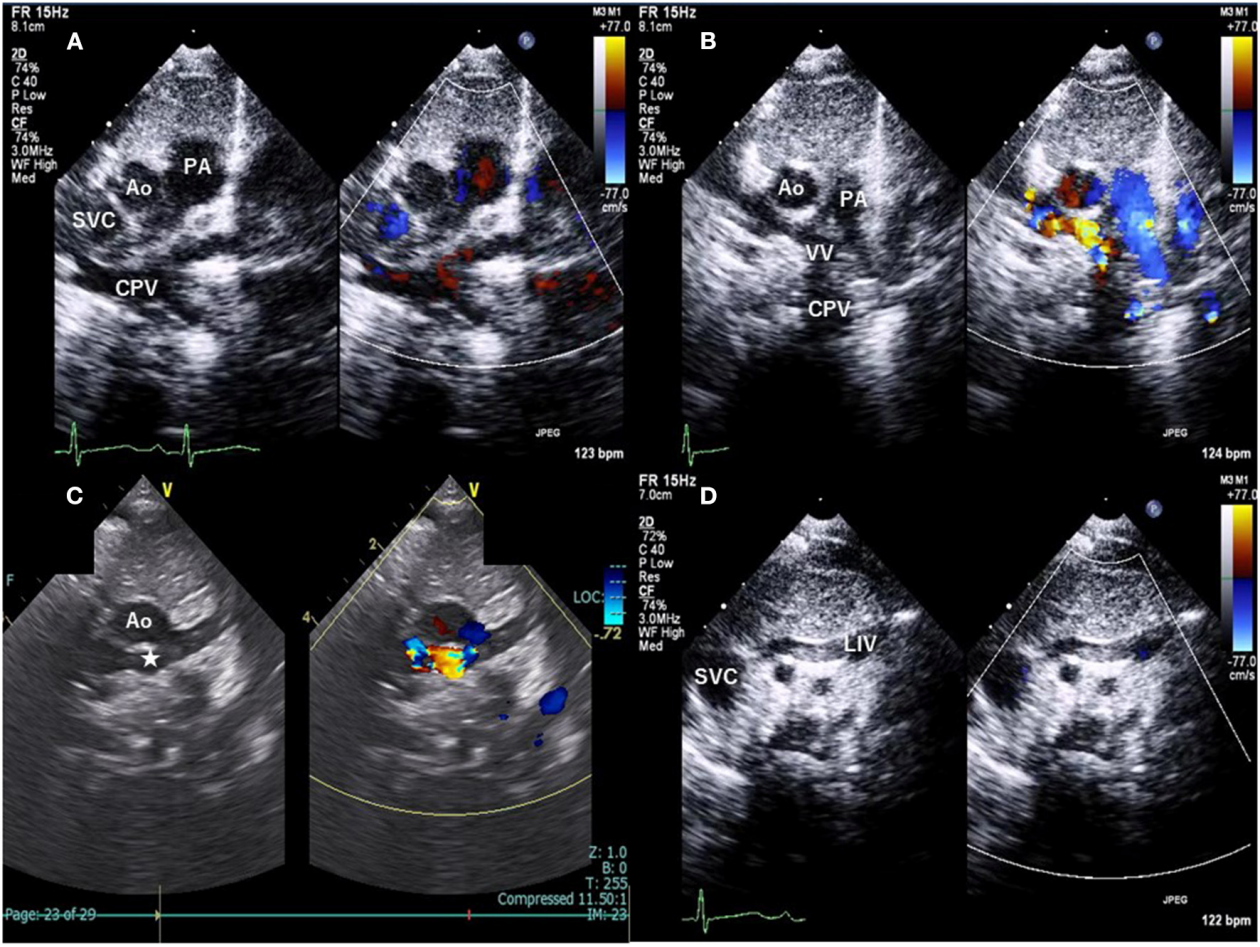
Suprasternal notch view of transthoracic echocardiography with color Doppler. (A) The pulmonary venous confluence (CPV) posterior to the left atrium. (B) The vertical vein (VV) originating from the CPV. (C) The anomalous vessel (asterisk) flowing from left to right behind the ascending aorta. (D) The left innominate vein in its normal position is not so dilated. Ao indicates aorta; PA, pulmonary artery; SVC, superior vena cava; LIV, left innominate vein.
